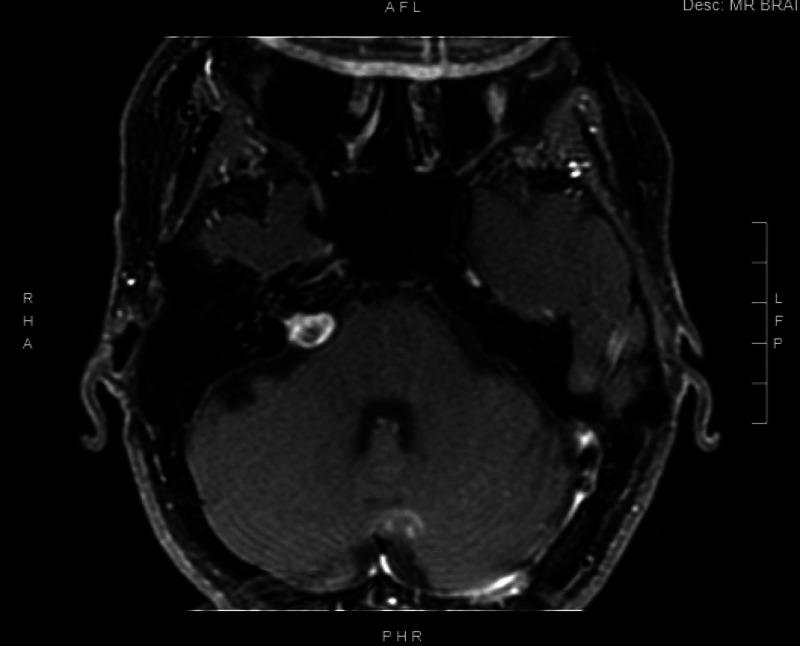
Post-stereotactic radiosurgery (SRS) MRI Axial T1 post-contrast image demonstrating the right vestibular schwannoma with a central necrosis, after SRS using Gamma Knife.
